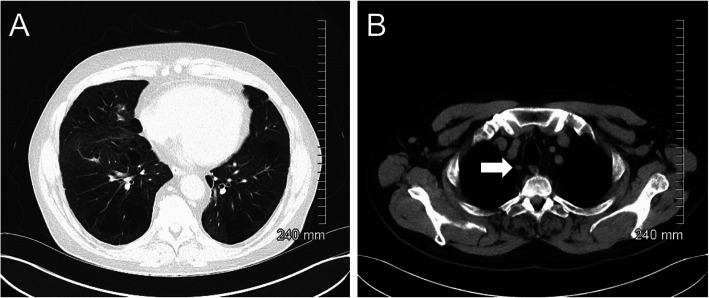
Initial CT thorax demonstrated no acute pulmonary infiltrates or lymphadenopathy (a). A morphologically normal ATS station 2R lymph node is highlighted by the white arrow (b)
